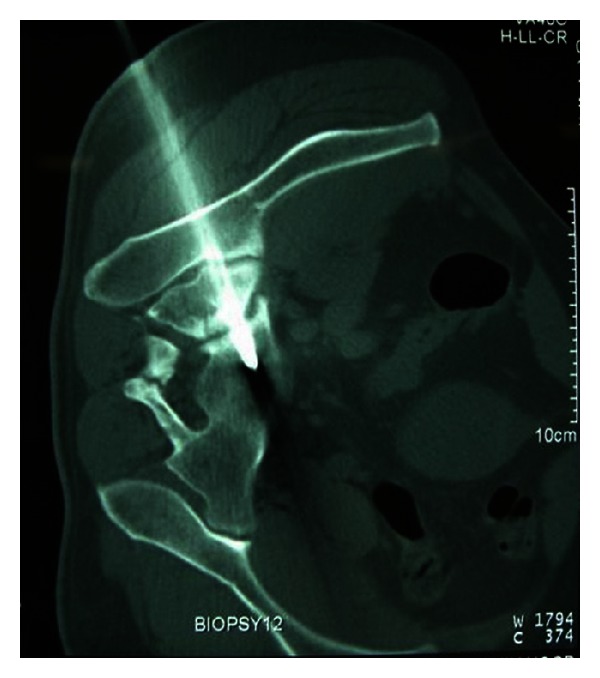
A CT image of the pelvis, showing a guide pin advanced through the iliac wing across the sacroiliac joint to the mid-body of S1.
